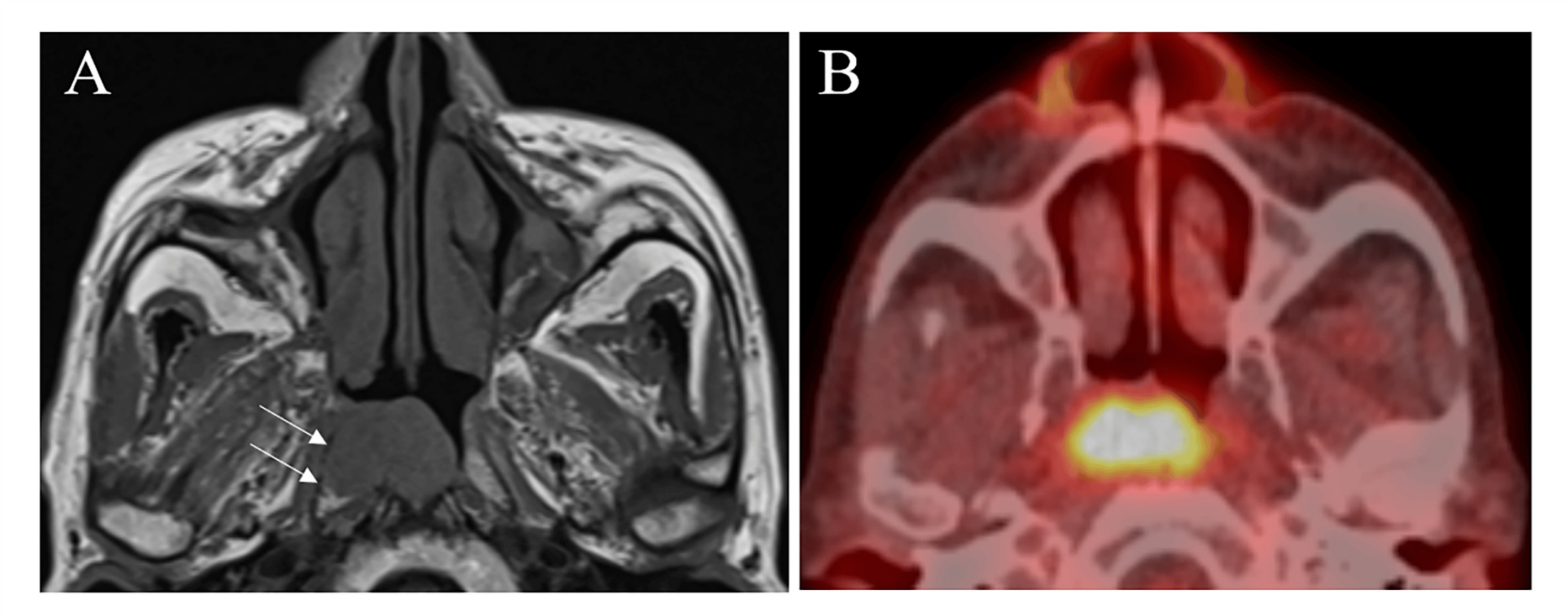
Imaging studies at the initial examination. (A) T1-weighted MRI showed the tumor was in contact with the right Eustachian tube (arrow). (B) PET-CT showed FDG uptake with a maximum standardized uptake value (SUVmax) of 9.8 in the tumor.
